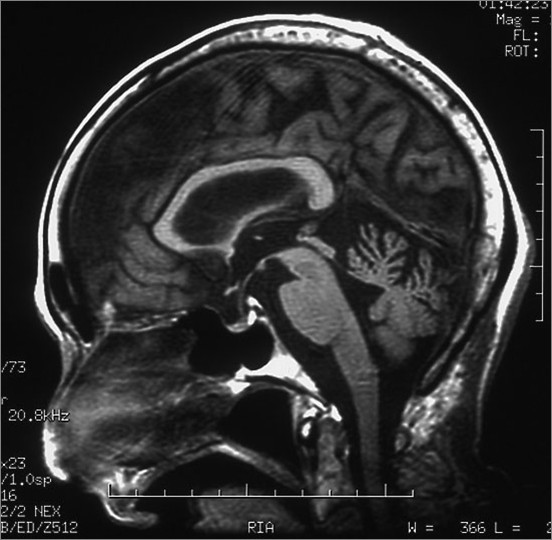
Brain MRI. Conventional brain MRI showed mild cerebellar atrophy, which seemed to be consistent with age.
